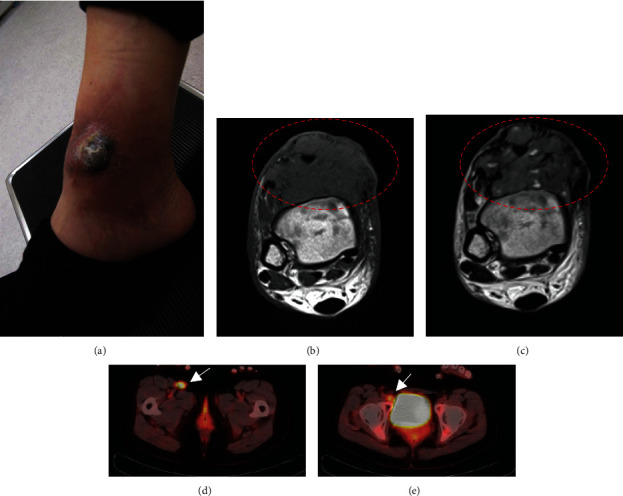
Local recurrence and lymph node metastasis at the initial visit. (a) Visual appearance of the ankle region during the patient's first visit to our hospital. (b) Axial image of the ankle region acquired using T1-weighted MRI. (c) Axial image of the ankle region acquired using T2-weighted MRI. (d) Axial image focused on the inguinal iliac lymph node acquired using positron emission tomography (PET). (e) Axial image focused on the external iliac lymph node acquired using PET.
